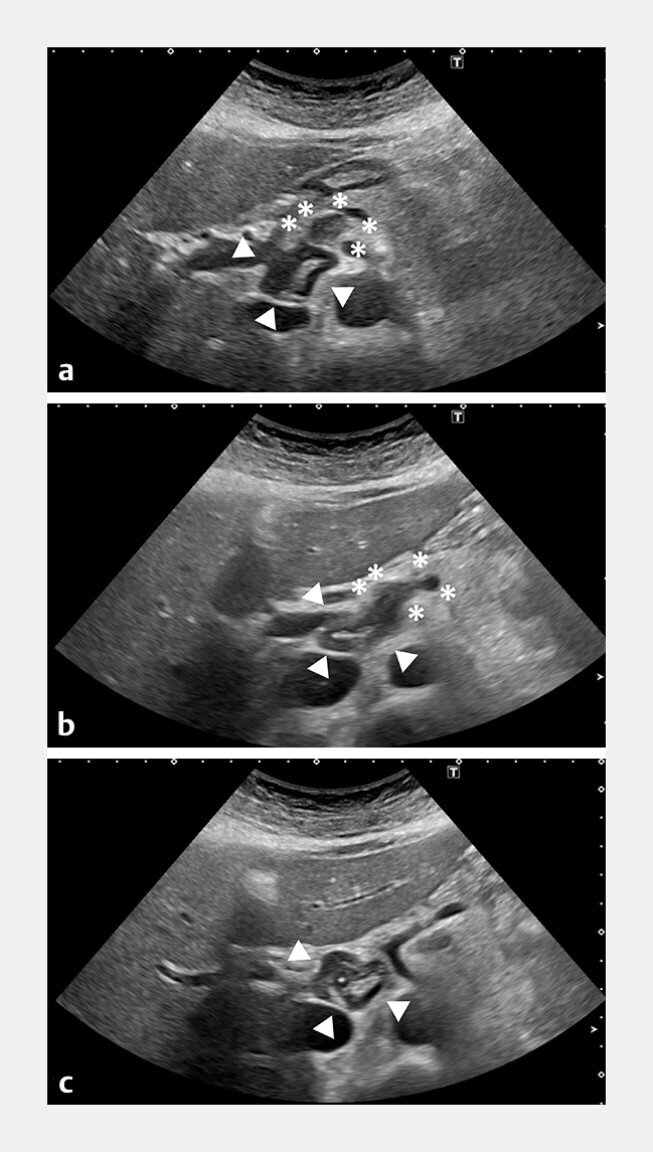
Transabdominal ultrasonography at 6 months after initial presentation.
